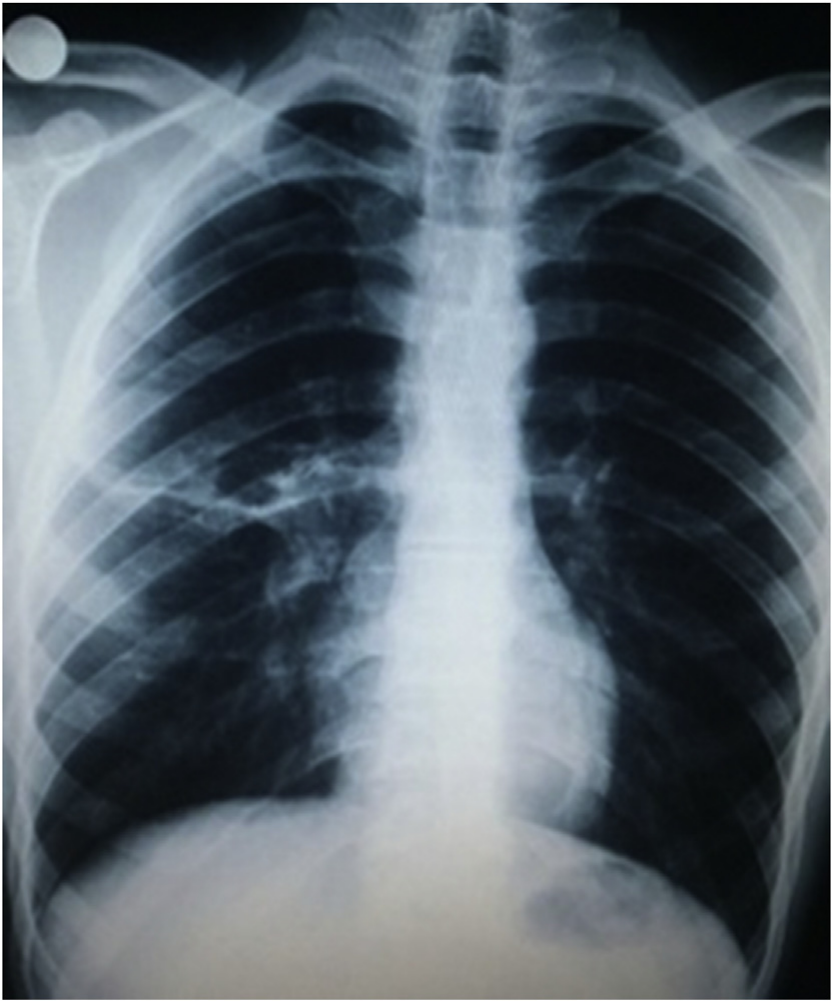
Postoperative view of the Chest X-Ray.
